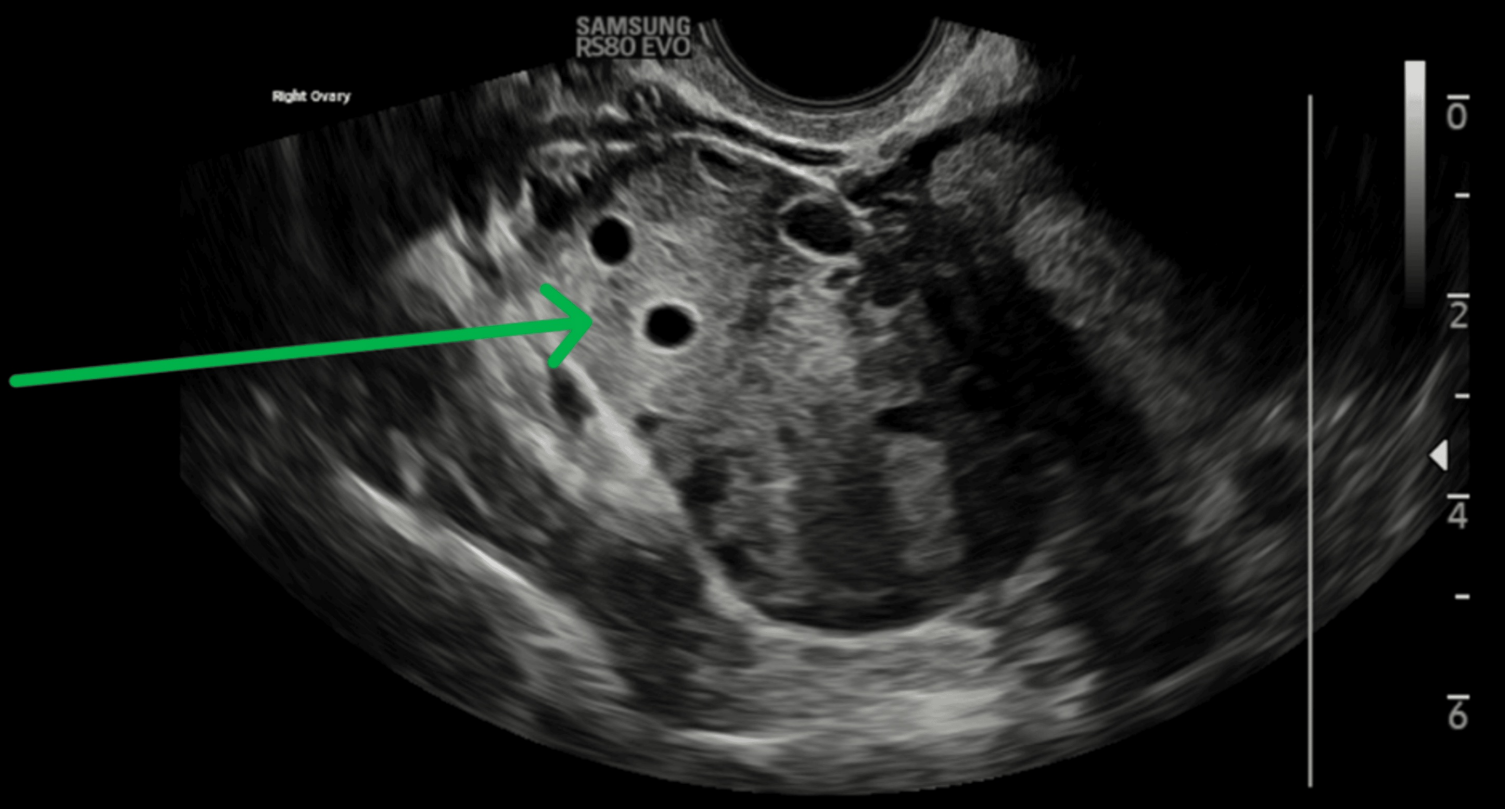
TVUS showing a right ovary measuring 60 mL with classic PCOS morphology: peripherally arranged follicles (arrow). TVUS, Transvaginal ultrasound; PCOS, Polycystic ovary syndrome
